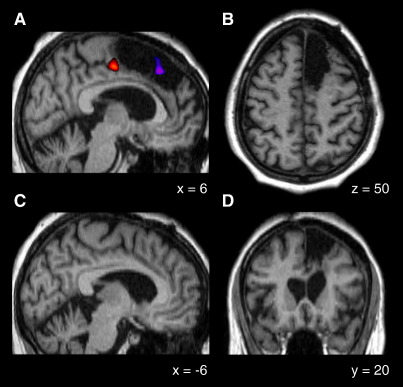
Lesion localisation. (A) Activation in the region of the SEF – a marker for the rostral extent of the SMA – superimposed on T1-weighted anatomical scan normalised in MNI space (orange). The purple area identifies the location of the rostral pre-SMA region which we have previously shown to be activated by changing oculomotor plans during conflict. (B–D) Sagittal, axial and coronal anatomical slices showing the extent of the lesion.
